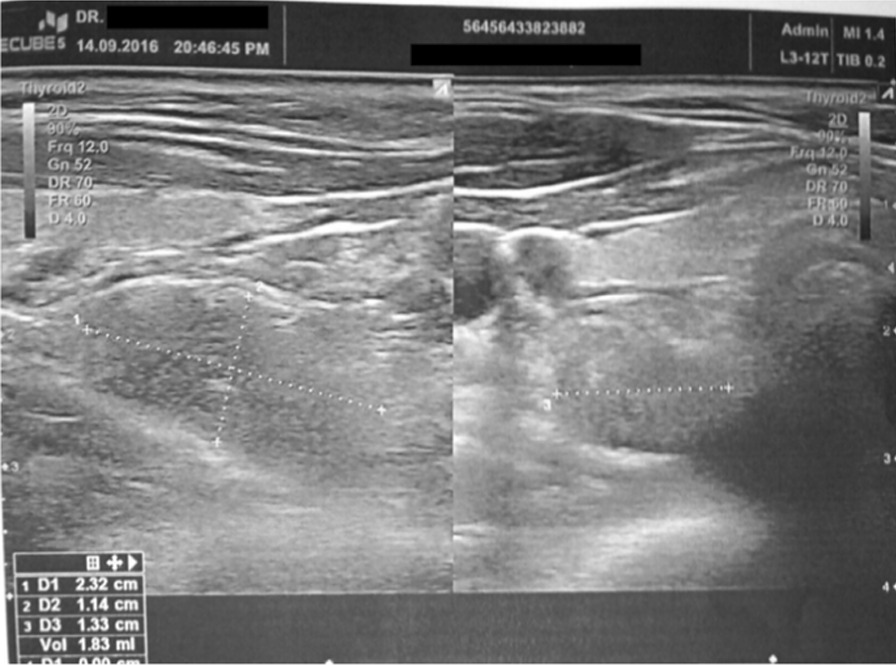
Thyroid ultrasound revealing an inferior right-sided nodule, indicative of parathyroid adenoma
